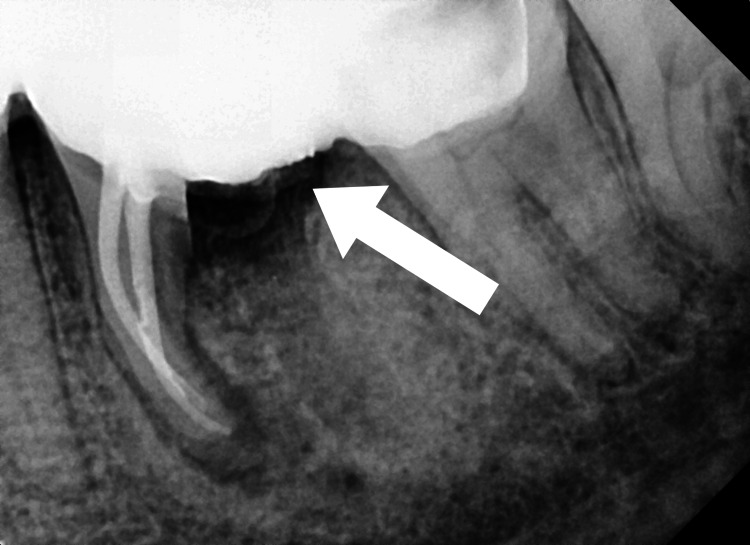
Radiograph showing follow-up at six months for the tooth number 36. The periapical region associated with the mesial root of tooth number 36 displayed a healing lesion. An incomplete resolution of the periapical lesion associated with this root was observed
